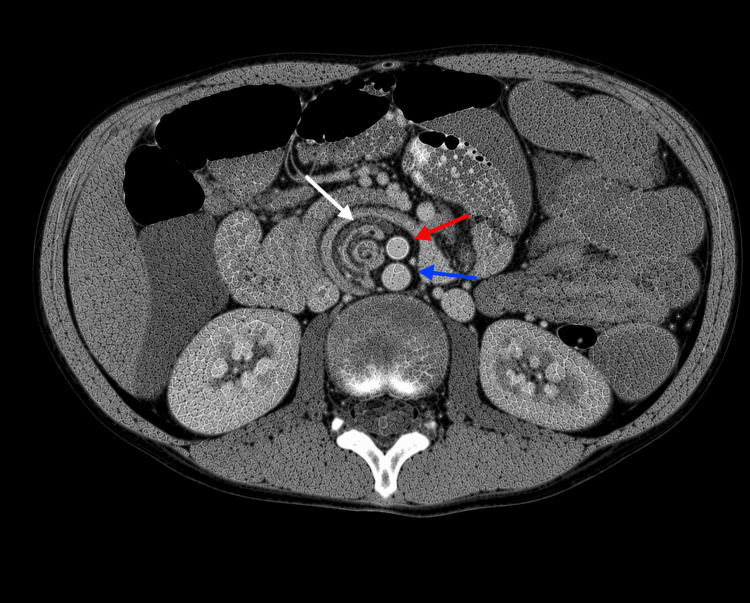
Contrast-enhanced computed tomography (CECT) scan of the abdomen demonstrating the classical whirlpool sign in midgut volvulus secondary to intestinal malrotation. Twisting of the mesenteric vessels around the superior mesenteric artery (SMA) is seen at the mesenteric root. The red arrow indicates the SMA, the blue arrow indicates the superior mesenteric vein (SMV), and the white arrow indicates twisting of the mesenteric vessels surrounding the mesenteric root.
